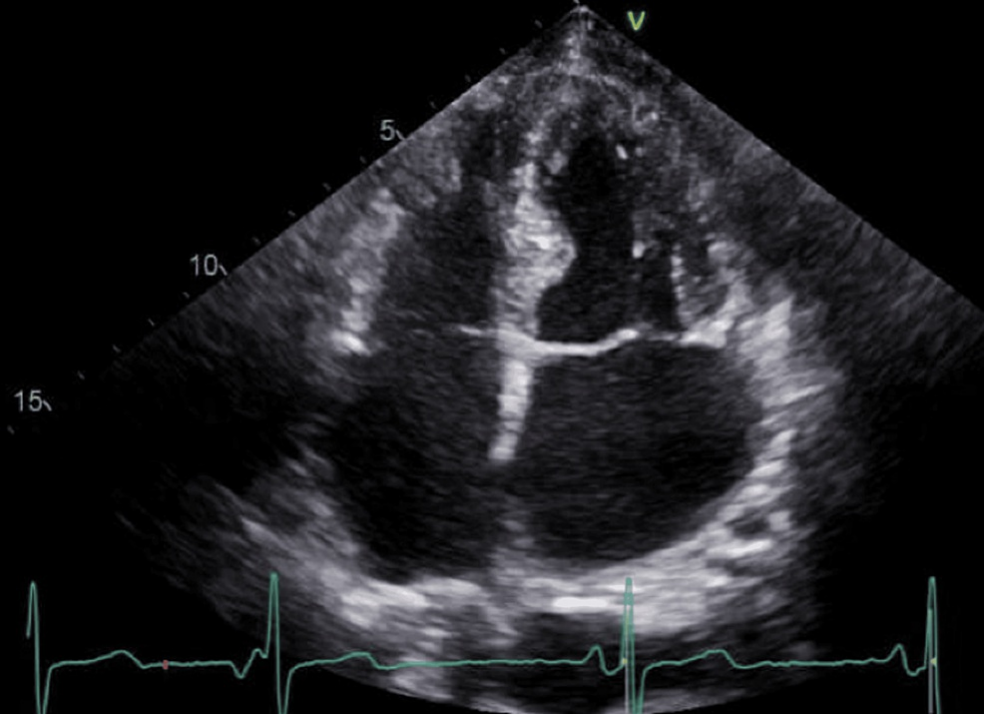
Post-treatment TTE showing resolution of previously noted right atrial mass. TTE: transthoracic echocardiogram.
